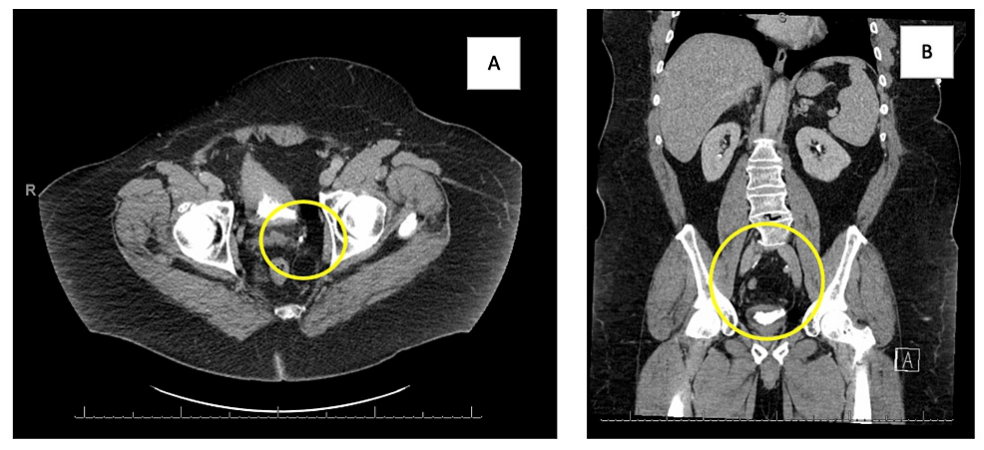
Abdominal CT (A) a filling defect on the left side of the bladder consistent with a transitional cell carcinoma. After surgical resection and mitomycin C treatment, (B) the resolution of the filling defect.
